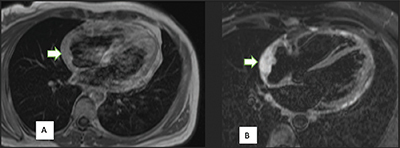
Right atrium angiosarcoma, a broad-based mass in the right atrium associated with thickening of the pericardium, is predominantly isointense to the myocardium on the T1-weighted image (arrow) (A). On the T2-weighted image (B), the mass shows a typical hyperintensity (arrow).
